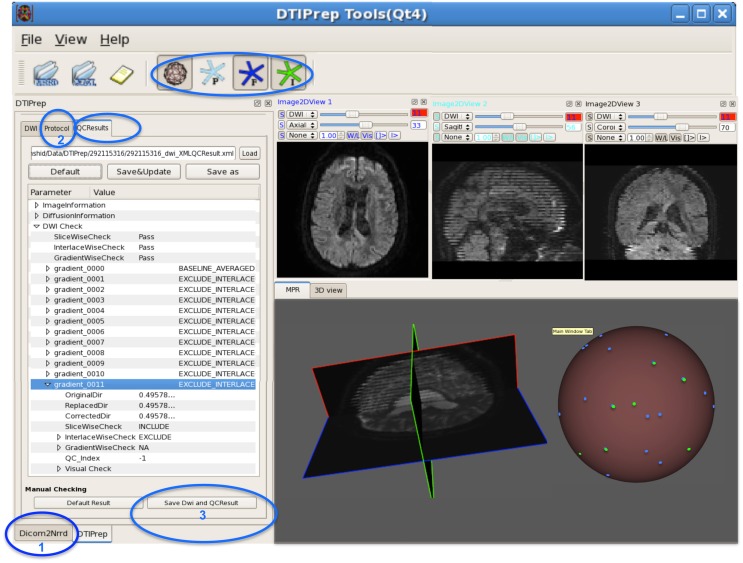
DWI-based QC results using DTIPrep through three steps. (1) converting the DWI image from DICOM to NRRD format, (2) loading the protocol and running the software, and (3) if necessary, visual checking and saving the final DWI dataset. In this example, gradient #11 suffers from intensity artifact and is excluded. The sphere shows a 3D view of the gradient distribution before (blue dots) and after running DTIPrep (green dots, visualized on top of the blue dots), respectively. In this particular example, a large number of DWI's were excluded (missing green dots on the 3D sphere). The 3D sphere also reveals a highly non-uniform distribution of the input diffusion gradient, indicating a non-optimal acquisition protocol.
